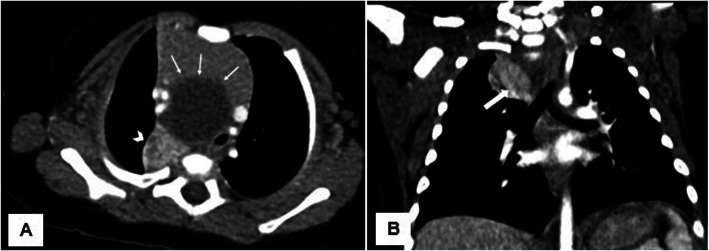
Axial (a) contrast-enhanced CT of the chest of a 3-month-old male infant showing a median cystic mass (arrows) causing tracheal deviation and compression, as well as a mass effect on the more anterior thymus, was pathologically demonstrated to be a bronchogenic cyst. A posterior right upper lobe hyperdense consolidation (arrowhead) with anomalous venous drainage to the distal SVC (block arrow), consistent with pulmonary sequestration, is better demonstrated on the coronal image (b)
